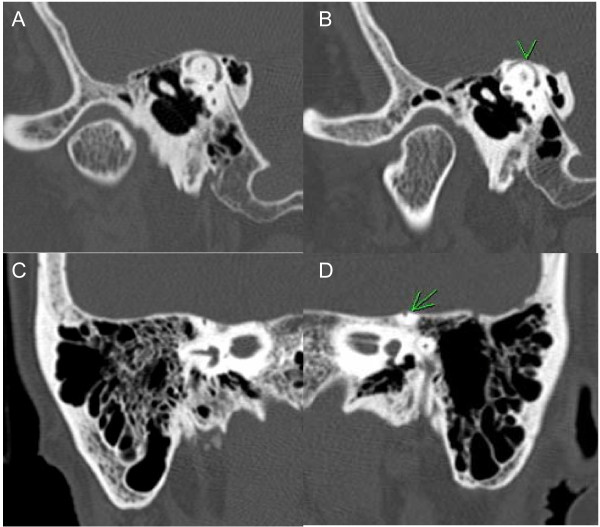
High-resolution multi-detector CT of the temporal bone was performed with 0.625 mm slices and reformations of the SSC along the longitudinal axis (Pöschl view) and transverse axis (Stenvers view). To measure the extent of dehiscence, a straight line was drawn subtending the arc of the SSC and rounding to the nearest 0.5 mm in the Pöschl view [3]. In patient 1, the petrous bone overlying the right SSC was eroded, but without dehiscence, as seen on the (A) Pöschl view and (C) Stenvers view. However, there was dehiscence of the left SSC as seen on the (B) Pöschl view (arrowhead) and (D) Stenvers views (arrow).
